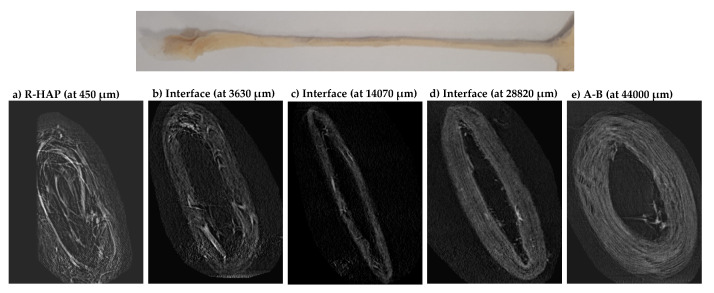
Micro CT images of the cross-sections acquired along the length of tubular scaffold from (a) the bone part (R-HAP), to (e) the tendon part (A-B), throughout (b), (c), and (d) the interface area.
